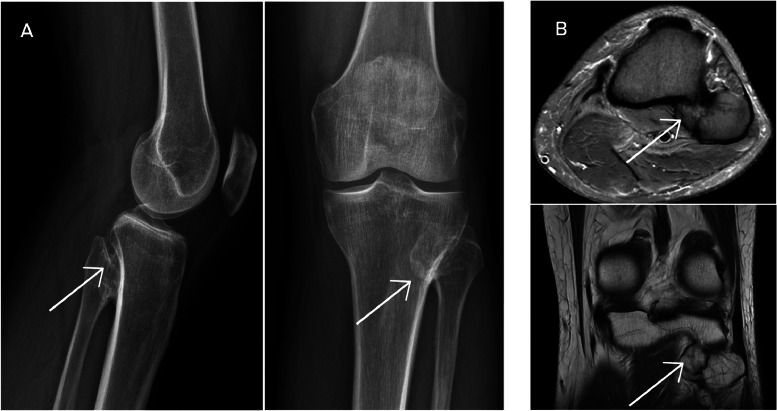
(A) Lateral, anteroposterior, and posteroanterior plain radiographs of the right knee showing an enlarged fibular head. (B) Axial and coronal MRI images confirming the enlarged fibular head without dislocation or subluxation.
